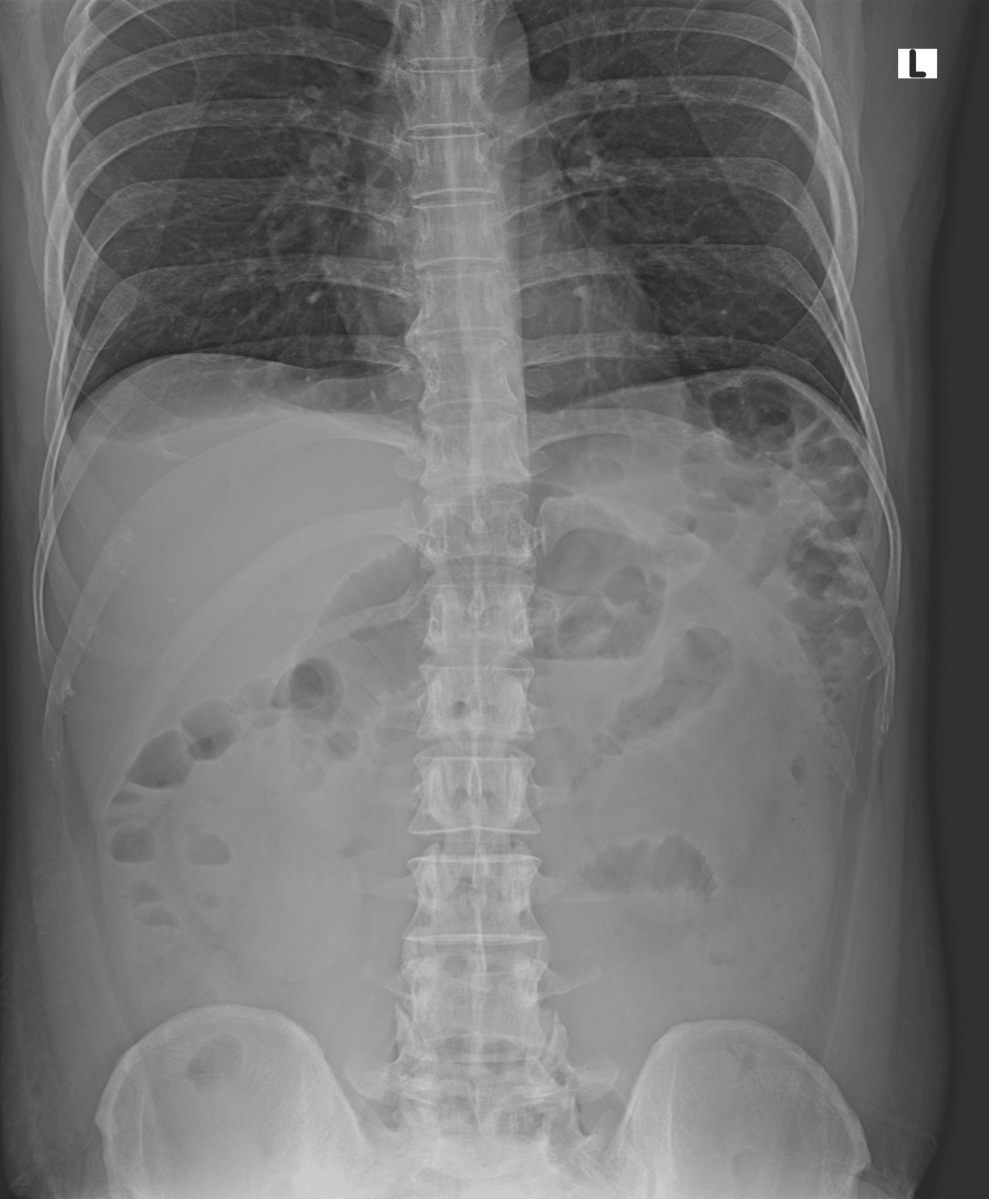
X-ray abdomen-erect showing nonspecific changes
